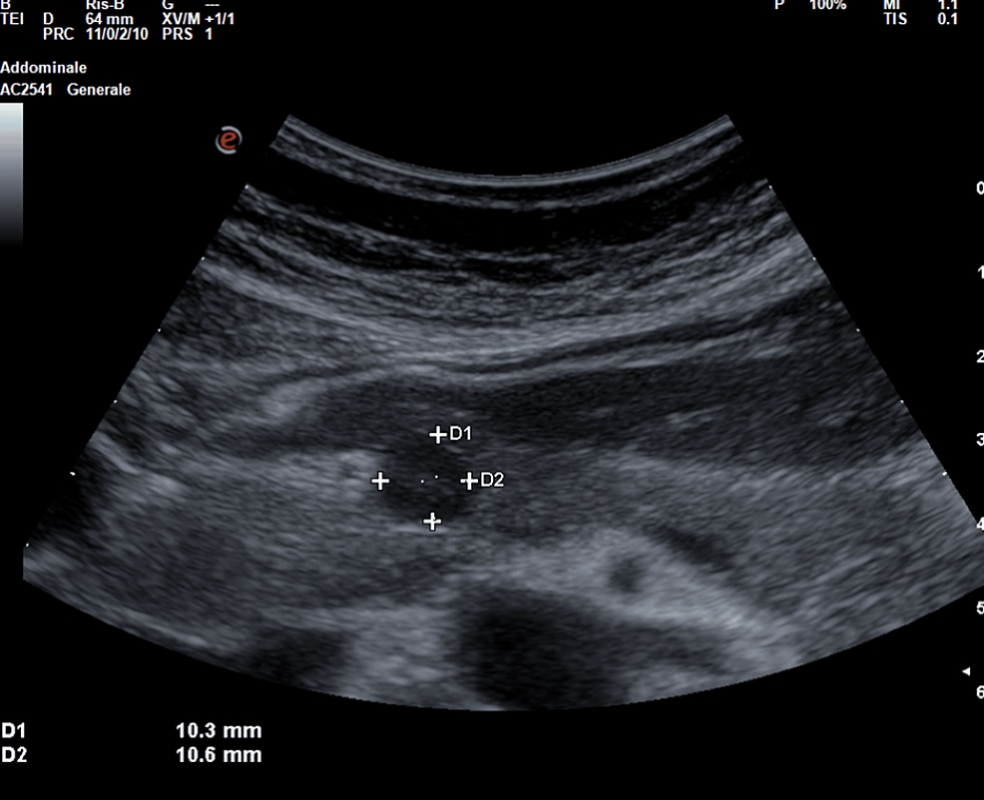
Bedside US from our patient conducted with Esaote MyLab Omega model with abdominal probe and preset showed a well-defined rounded lesion in the isthmic region of the pancreas, hypoechoic compared to the surrounding pancreatic parenchyma, of dimensions 11x10 mm. US: ultrasonography
